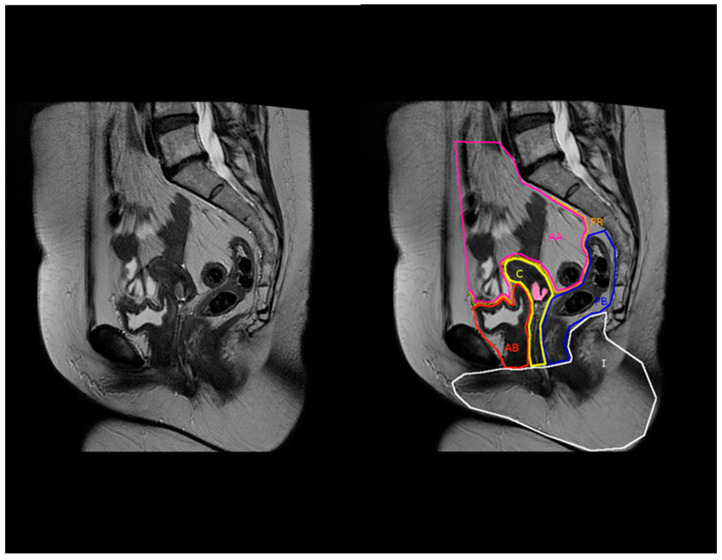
Conventional (left) and compartment-based (right) magnetic resonance imaging, sagittal T2-weighted image. AA: anterior above peritoneal reflection compartment, PR: peritoneal reflection compartment, AB: anterior below peritoneal reflection compartment, C: central compartment, PB: posterior below peritoneal reflection compartment, I: inferior compartment. Cervical tumor is highlighted in pink and extends towards the anterior below peritoneal reflection compartment (AB).
